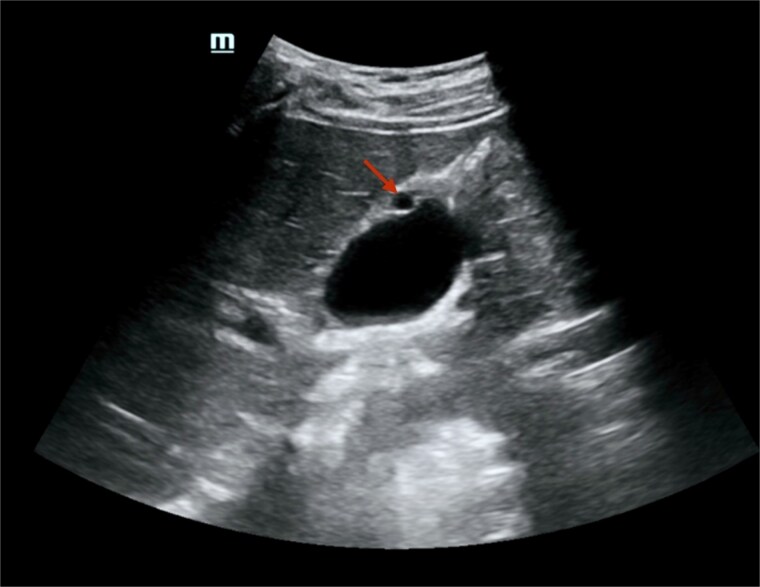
Abdominal ultrasound showed a well-defined, round, anechoic lesion within the gallbladder wall, measuring about 7 mm, likely representing a foregut cyst of the gallbladder. There was no peri-cholecystic edema, obvious shadowing stones, or increased wall thickness.
